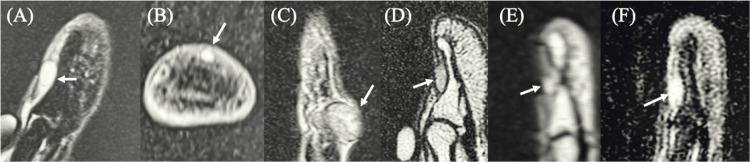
Representative MRI findings of subungual glomus tumors. Representative MRI findings of subungual glomus tumors in six patients who were correctly diagnosed preoperatively. Typical lesions are shown as small, well-defined nodules with low signal intensity on T1-weighted images and high or isointense signal intensity on T2-weighted and short tau inversion recovery (STIR) sequences. For each case, the image that most clearly demonstrated the lesion was selected from available MRI sequences and imaging planes. The optimal sequence and imaging plane for lesion visualization varied among cases. (A) T2-weighted, sagittal view, (B) T1-weighted, axial view, (C) T2-weighted, sagittal view, (D) T2-weighted, sagittal view, (E) T2-weighted, sagittal view, and (F) STIR, sagittal view.
